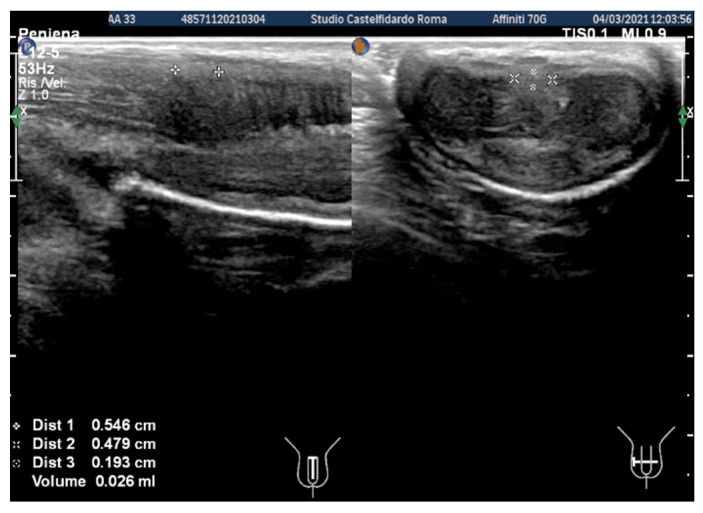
Ultrasonography of the penis after the second treatment cycle (longitudinal and transverse views).
